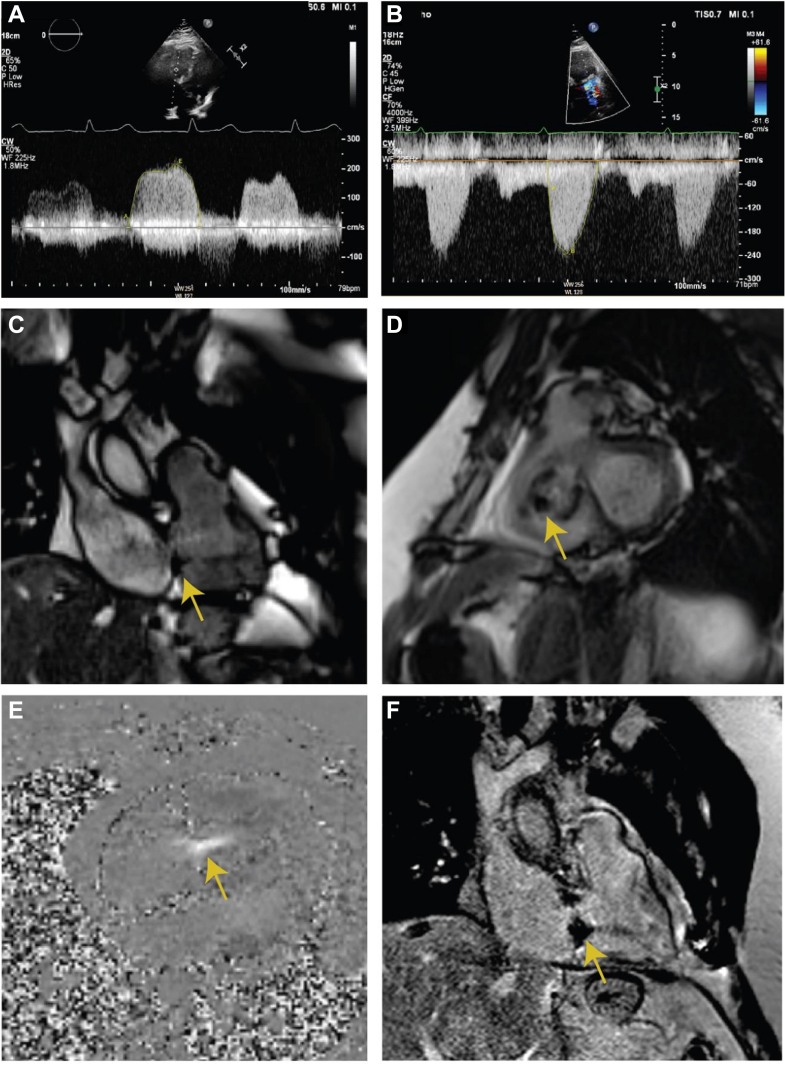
Early Follow-up Imaging (A) TTE of TV inflow showing severe stenosis (mean PG 12.2 mm Hg, max PG 18.6 mm Hg, VTI 86.6 cm). (B) TTE of PV transvalvular gradients remain unchanged at 10-month follow-up. (C and D) CMR demonstrating the 13 × 15-mm structure adherent to the inferior aspect of the TV leaflets, which protrudes into the RV. (E and F) CMR demonstrates no early or late gadolinium uptake. Structure density in keeping with thrombosis. CMR = cardiovascular magnetic resonance; PG = pressure gradient; PV = pulmonary valve; TTE = transthoracic echocardiography; TV = tricuspid valve; RV = right ventricular; VTI = velocity time integral.
